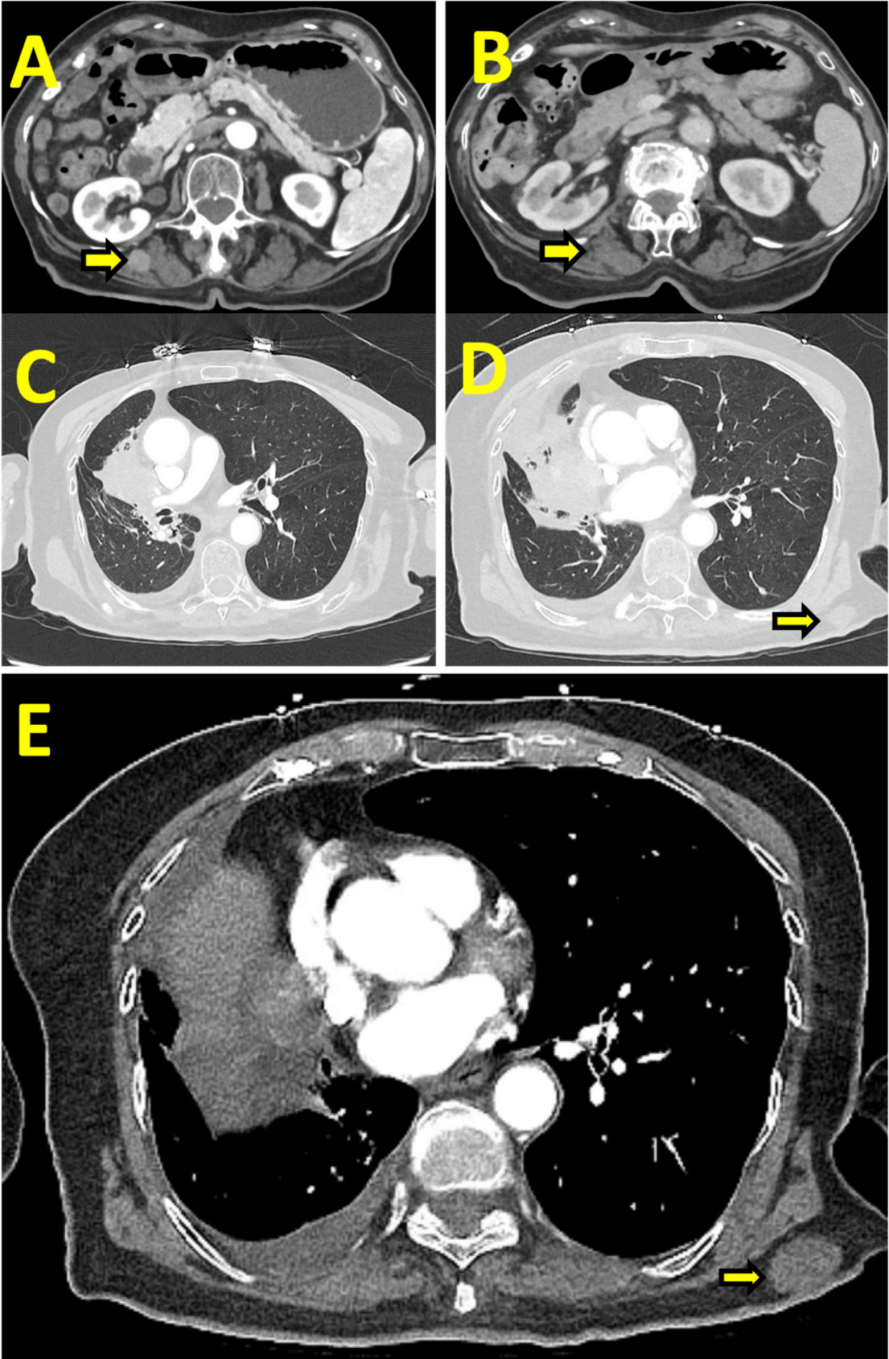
Axial CT chest images: (A) new soft tissue lesion between the right para‐spinal muscles (arrow); (B) decrease in size of the lesion post‐radiotherapy (arrow). (C and D) recurrence of right lung mass; (D and E) new subcutaneous lesion in left posterior chest wall (arrows).
